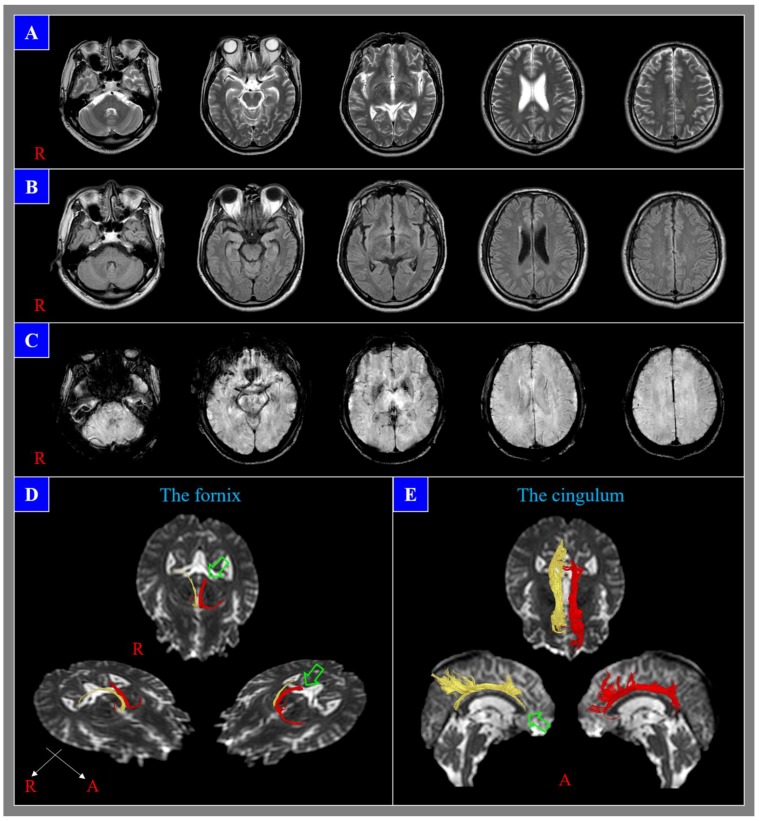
A 54-year-old male who showed loss of consciousness for seven days after a pedestrian–car accident does not show any lesions in (A) T2-weighted, (B) fluid attenuation inversion recovery (FLAIR), and (C) susceptibility-weighted imaging (SWI). However, diffusion tensor tractography results show neural injuries (discontinuations (green arrows): the left fornical crus and right anterior cingulum) of the fornix (D) and cingulum (E); these injuries are consistent with the subject’s cognitive impairment, which developed after head trauma.
